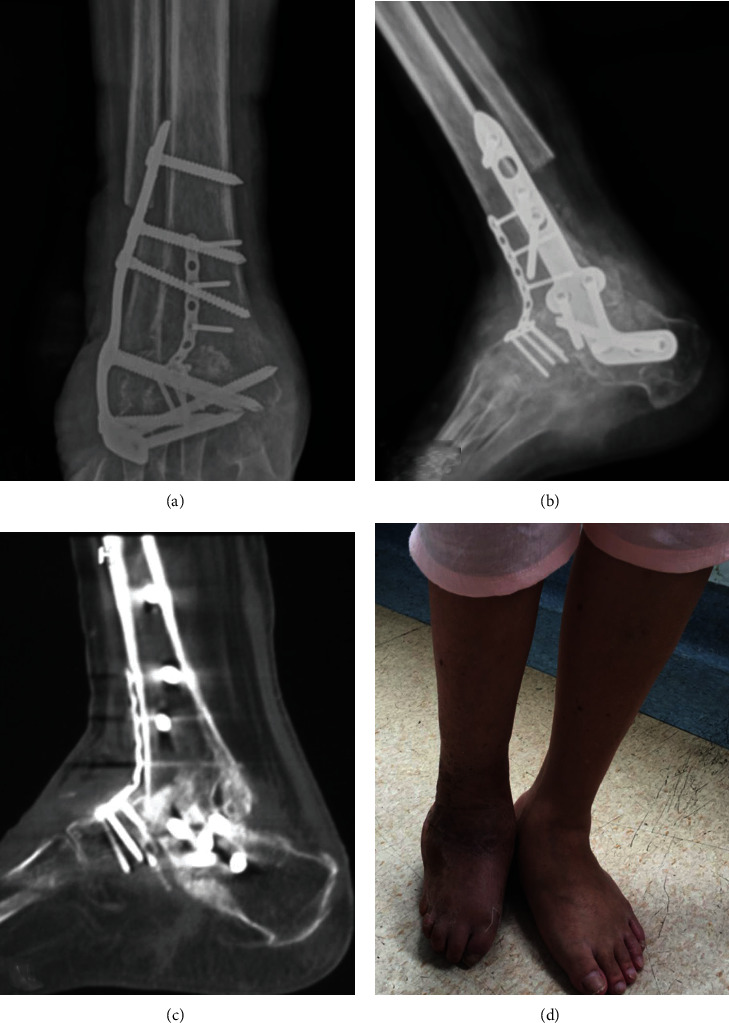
Postoperative imaging examinations and general appearance of right ankle joint. (a) and (b) Postoperative X-rays indicated that the osteotomy end was well aligned and the fixation effect was reliable. (c) At 12 weeks postoperatively, the osteotomy end obtained the bony fusion confirmed by CT scanning. (d) The general appearance of the right ankle joint at the last follow-up.
